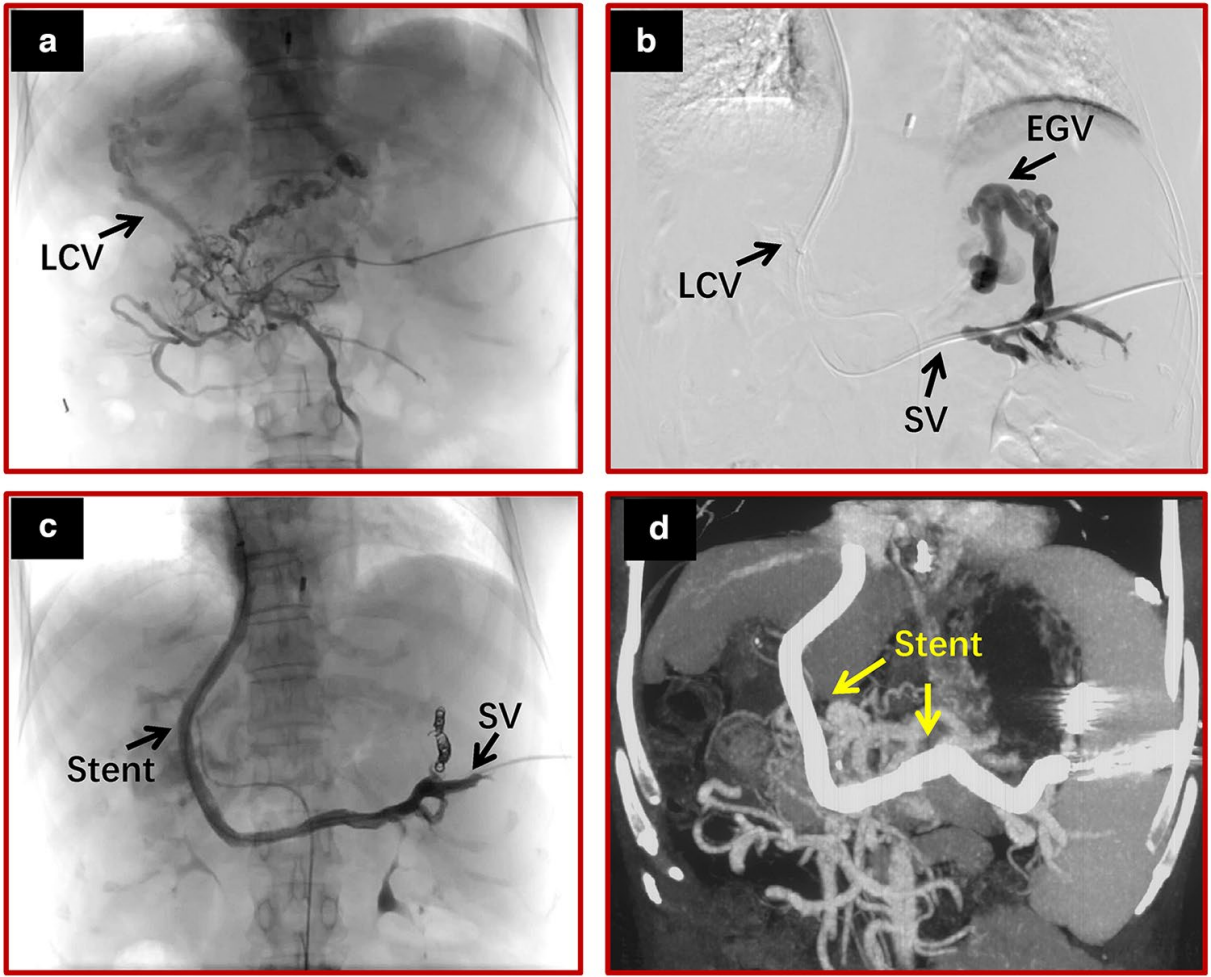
Using a collateral vessel that did not communicate with the variceal veins to establish a portosystemic shunt in a 57-year-old woman with cirrhosis and cavernous transformation. a Direct portography via a percutaneous transsplenic approach clearly showed that a large, cavernous collateral vessel could be used to establish a portosystemic shunt. b Direct splenoportography showed that the severe varicose veins did not communicate with the collateral vessel due to splenic vein thrombosis. c The collateral vessel was used to establish the portosystemic shunt. At the same time, the splenic vein was opened with a bare stent to make the varicose veins communicate with the collateral vessels, and the varicose veins were embolized. Direct splenoportography showed that the shunt was patent and that the varicose veins had disappeared. d The stents are shown on spiral enhanced CT with multiplanar reconstruction at 1 month after TIPS. *LCV* large collateral vessels, *EGV* esophageal and gastric varices, *SV* spleen vein
